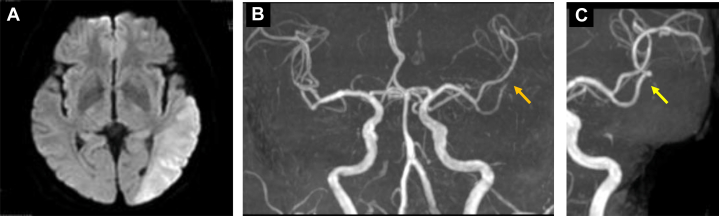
Magnetic Resonance Imaging Findings at the Time of Stroke Center Consultation (A) Magnetic resonance imaging revealed acute cerebral infarction in the left temporal lobe, and (B) magnetic resonance angiography demonstrated occlusion of the left middle cerebral artery M2 segment (orange arrow). (C) Follow-up imaging after 1 week of anticoagulation therapy showed recanalization of the occluded vessel (yellow arrow) without new brain infarction.
